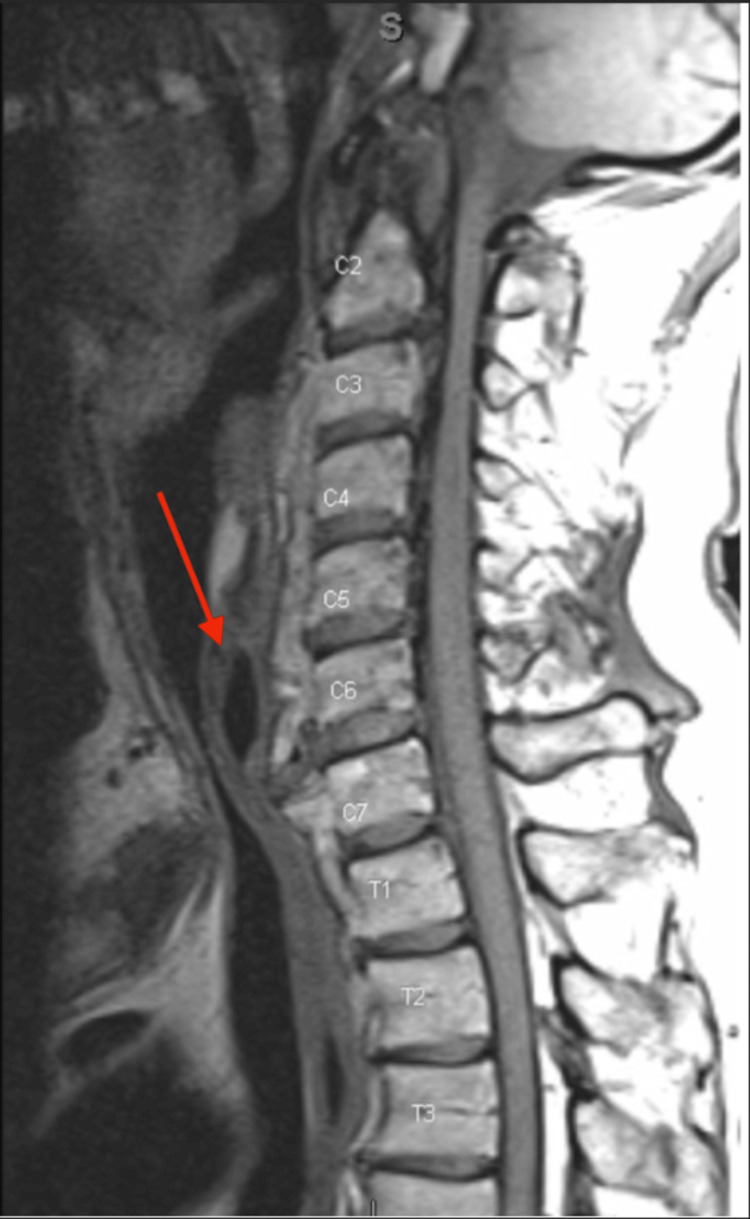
MRI of the cervical spine demonstrating extensive anterior osteophyte formation at C5–C7 (arrow).
